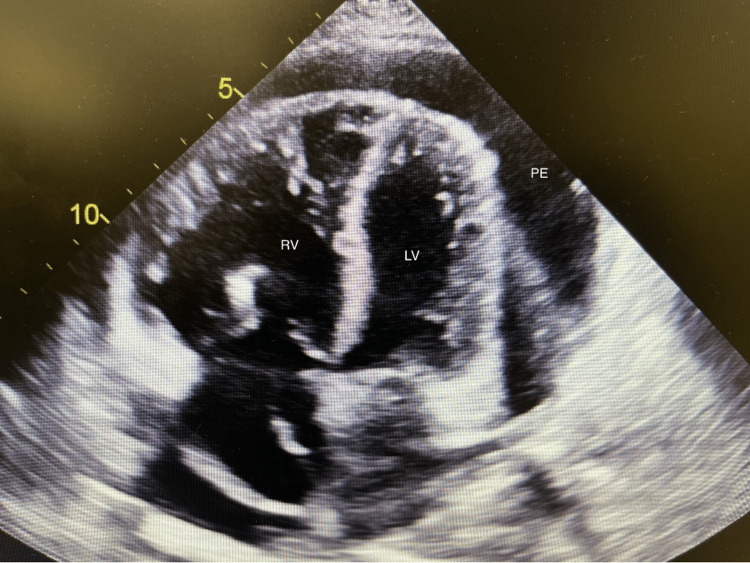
Apical view of the heart by 2D-echocardiogram with the presence of moderate to large pericardial effusion (PE) and underfilled left (LV) and right ventricle (RV) The left atrium is not shown because it collapsed.
